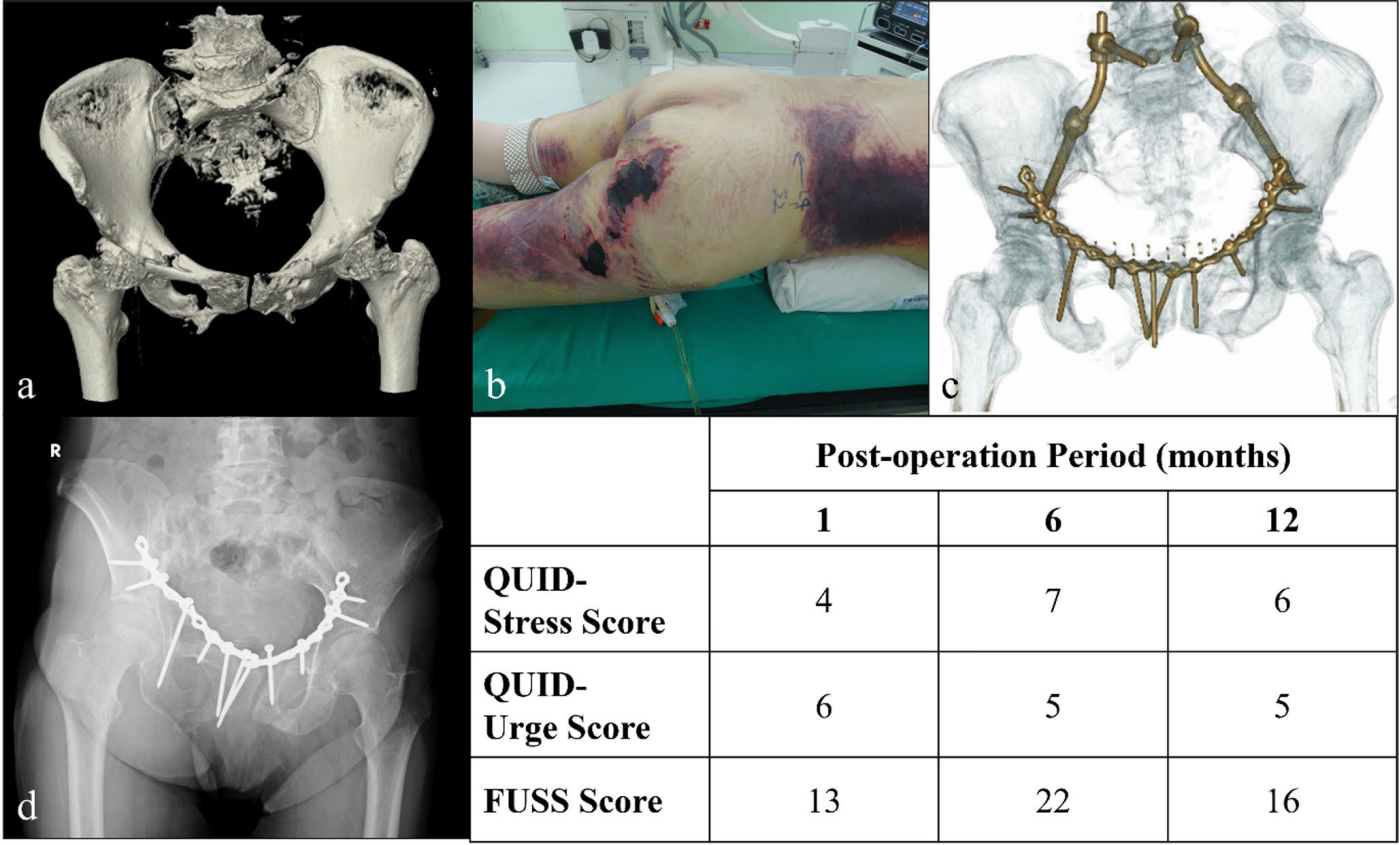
Case presentation of a 62-year-old woman with a VS pelvic ring injury (AO C3.3) sustained in a motor vehicle accident with related urinary sequelae evaluations. (a) and (b) Preoperative CT scans showing the injury and a Morel-Lavallée lesion involving the right buttock and the posterior thigh. (c) Postoperative CT image. (d) Follow-up image at 1 year postoperatively
